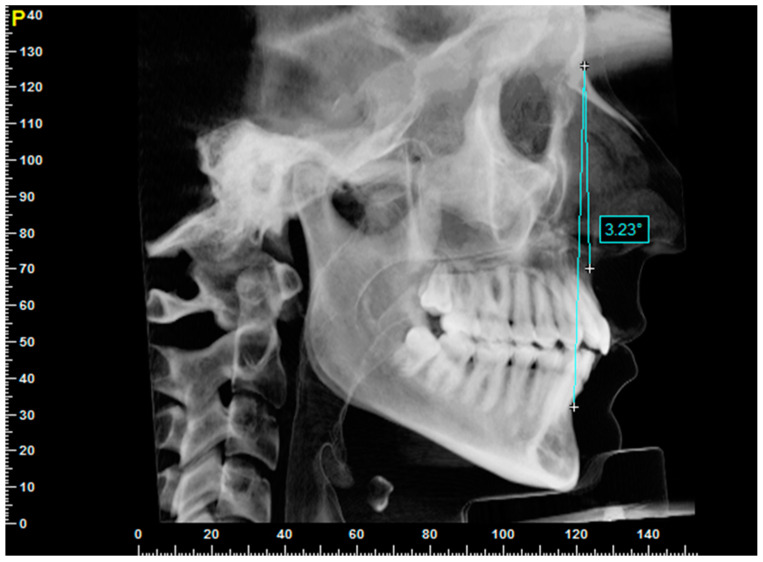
Ray-sum reconstruction of latero-lateral teleradiography from a CBCT examination.
